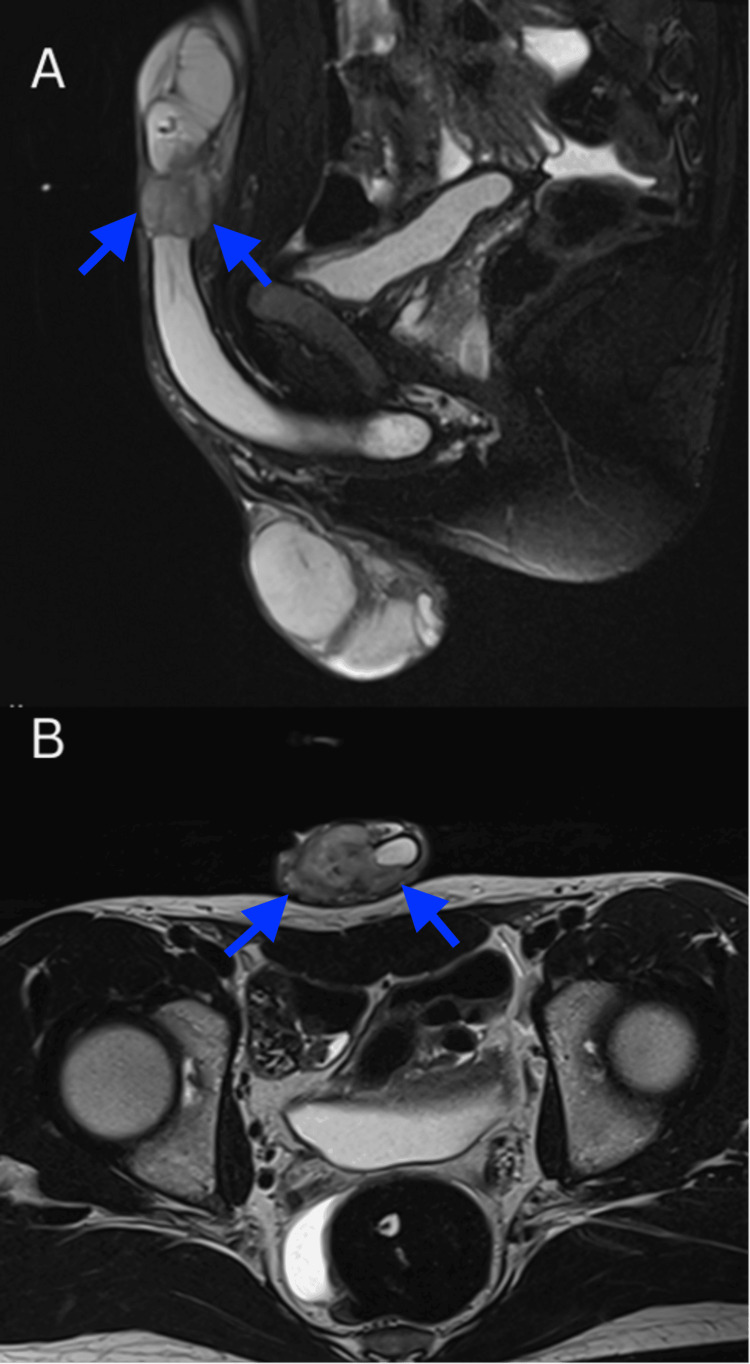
MRI with pharmacologically induced erection A. Sagittal MRI showing a penile mass involving the right corpus cavernosum. B. Axial slide exhibiting the partial extension to the left corpus cavernosum with sparing of the urethra MRI: magnetic resonance imaging
